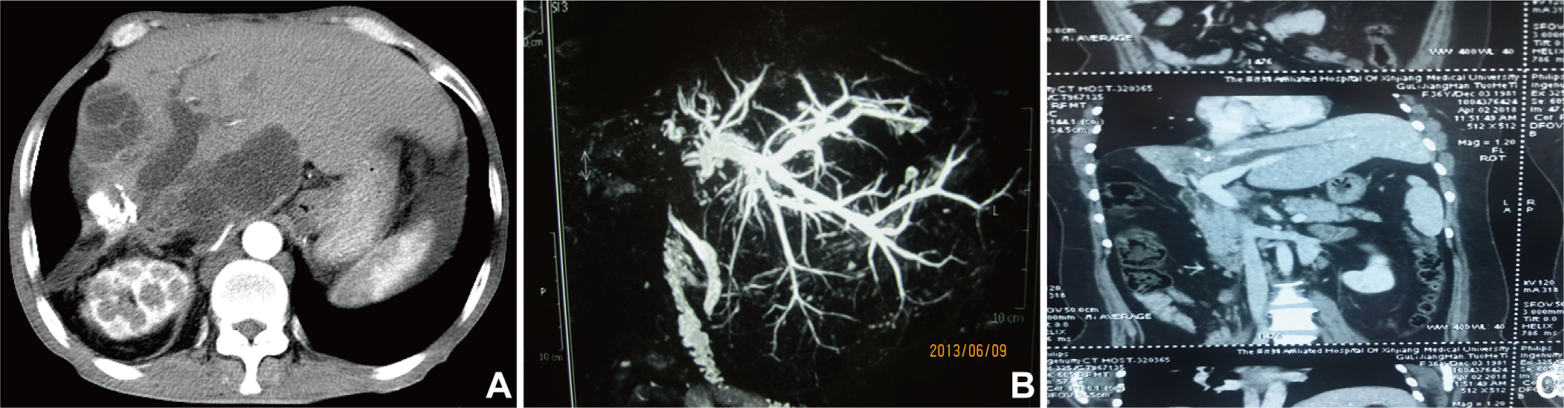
Representative imaging for type A hilar biliary duct stricture. (A) Abdominal enhanced CT demonstrating dilation of left hepatic duct caused by hilar calcification, hyperplasia of left hepatic lobe and two recurrent CE lesion. (B) Abdominal MRI indicating obvious dilation of left hepatic duct and discontinuity of portal hepatis. (C) Abdominal CT showing atrophy of right lobe and dilation of left hepatic duct.
